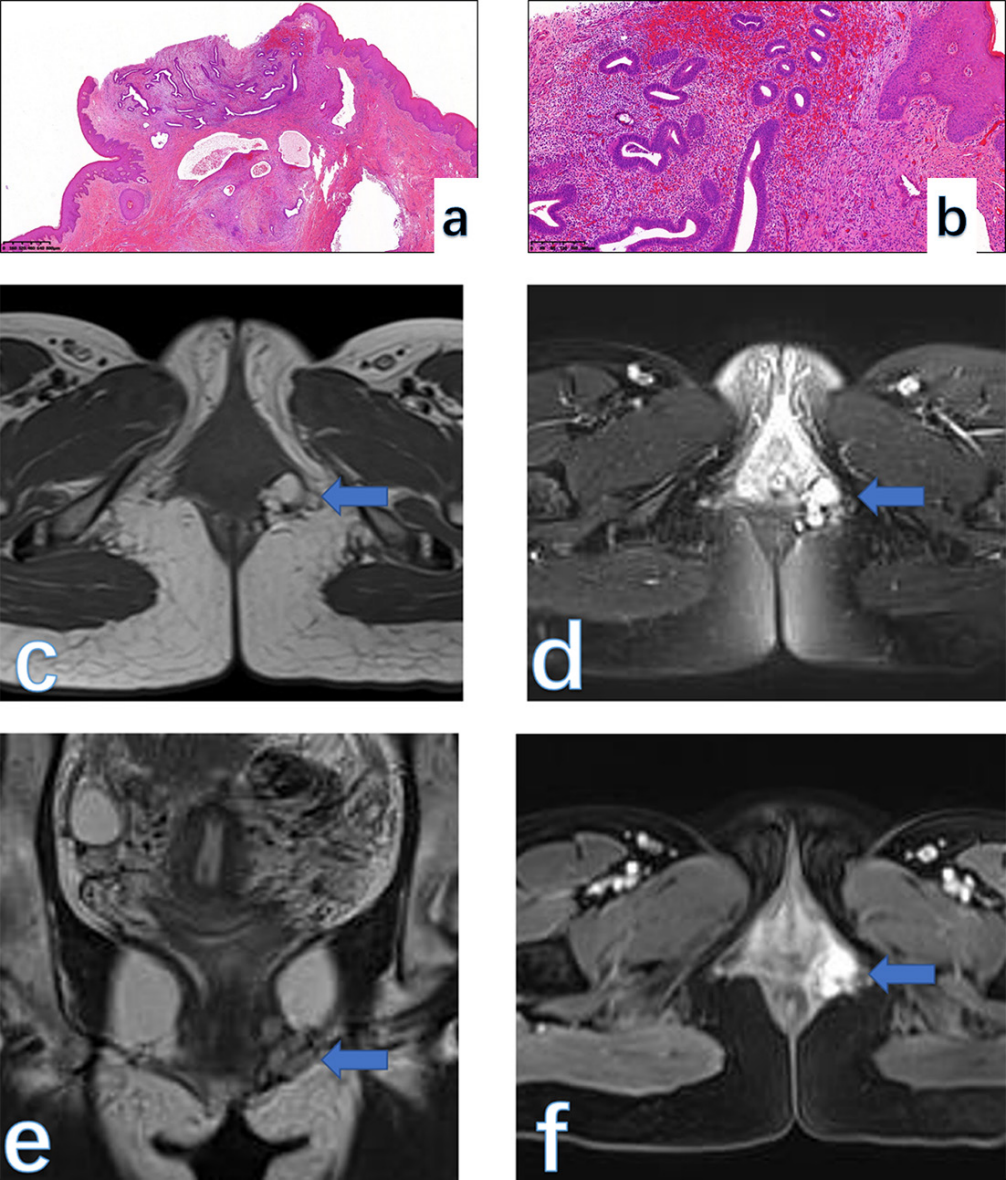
Endometrial glandular and stromal elements within the fibromuscular layer (a, HE × 25). Higher magnification of the field reveals endometrial glands surrounded by a hemorrhagic stroma within fibromuscular tissue (b, HE × 100). On MRI, in the left labium major, the multicystic lesion (arrow) with irregular margin displayed a high signal on both *T*
